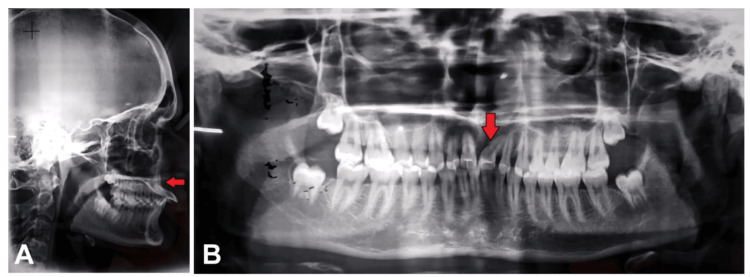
Pre-treatment radiograph. (A) Lateral cephalogram: Appreciate the red arrow, that represents upper incisor roots towards the palatal cortical plate due to severely proclined upper incisors. (B) Orthopantomogram: The red arrow depicts the distal inclination of the root with respect to tooth 21.
